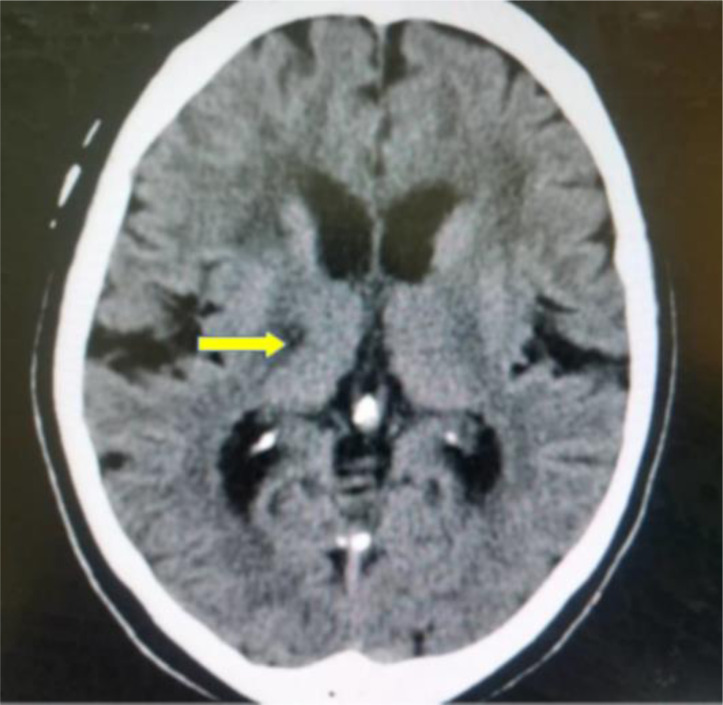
An axial non-enhanced CT scan of the brain showing an area of hypodensity at the right basal ganglia (small deep brain hypodensity) consistent with acute right basal ganglia infarct (shown with yellow arrow).
